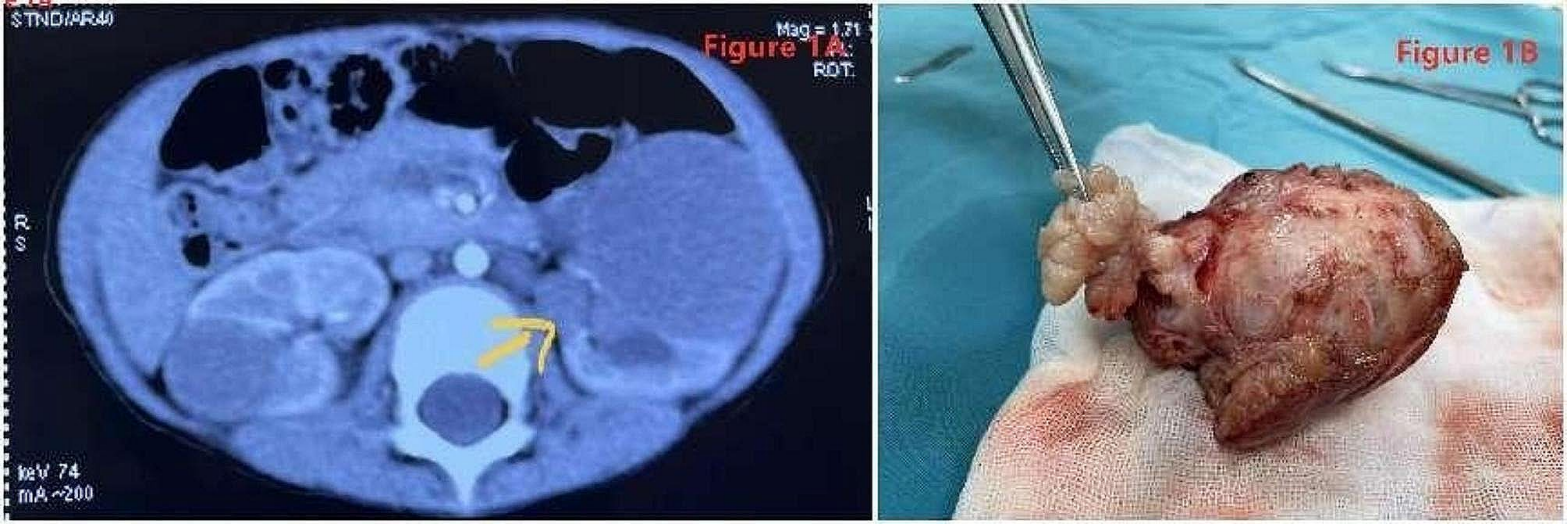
A Enhanced CT of bilateral wilms’ tumor with left tumor growing into the renal pelvis. B Postoperative gross pathology of the tumor, the renal pelvis mass can be seen intact, showing a grape-like growth pattern
